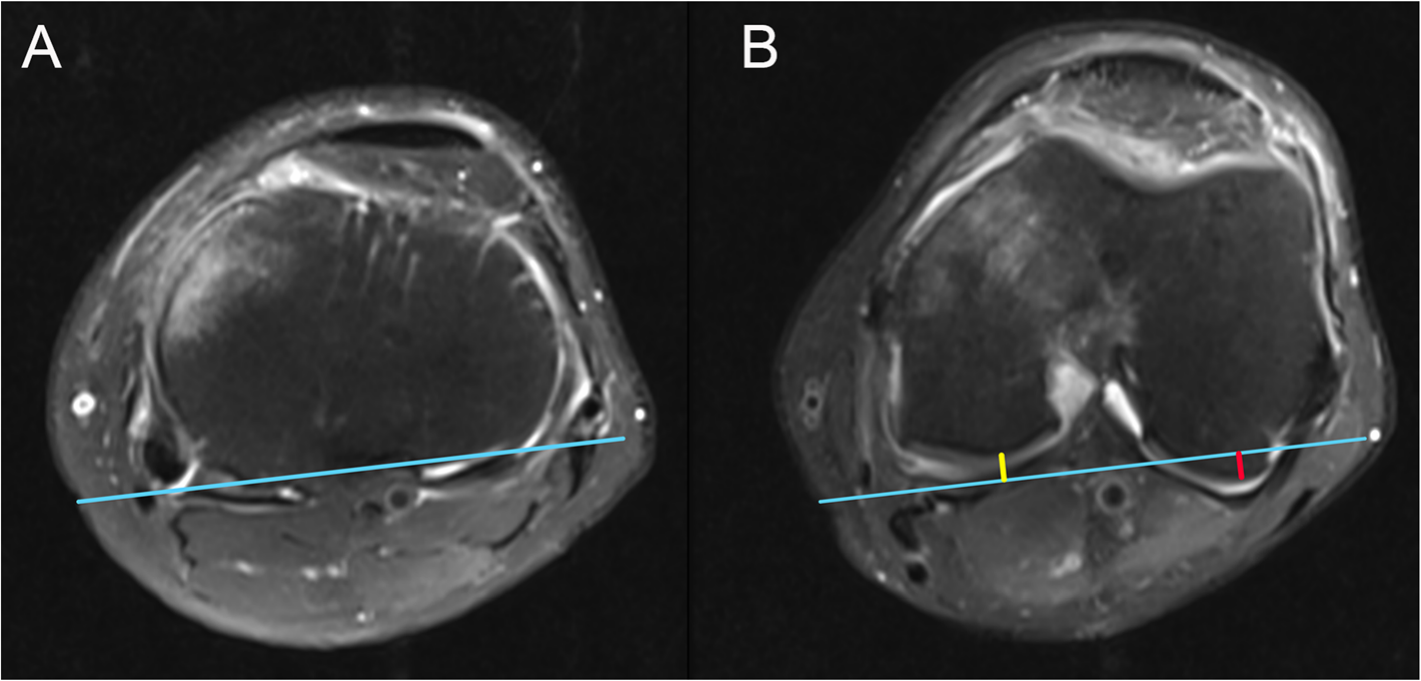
Measurements of the medial and lateral PATS. a Axial plane on MRI showing the line (blue) connecting the most posterior points of the tibial plateau. b After the most posterior points of femoral condyles are determined, the distance from both condyles to the blue line are measured. The length of the yellow line and the red line indicate the medial PATS and the lateral PATS, respectively. In this case, the value of medial PATS is negative (posterior translation), while the value of lateral PATS is positive (anterior translation). PATS, passive anterior tibial subluxation
